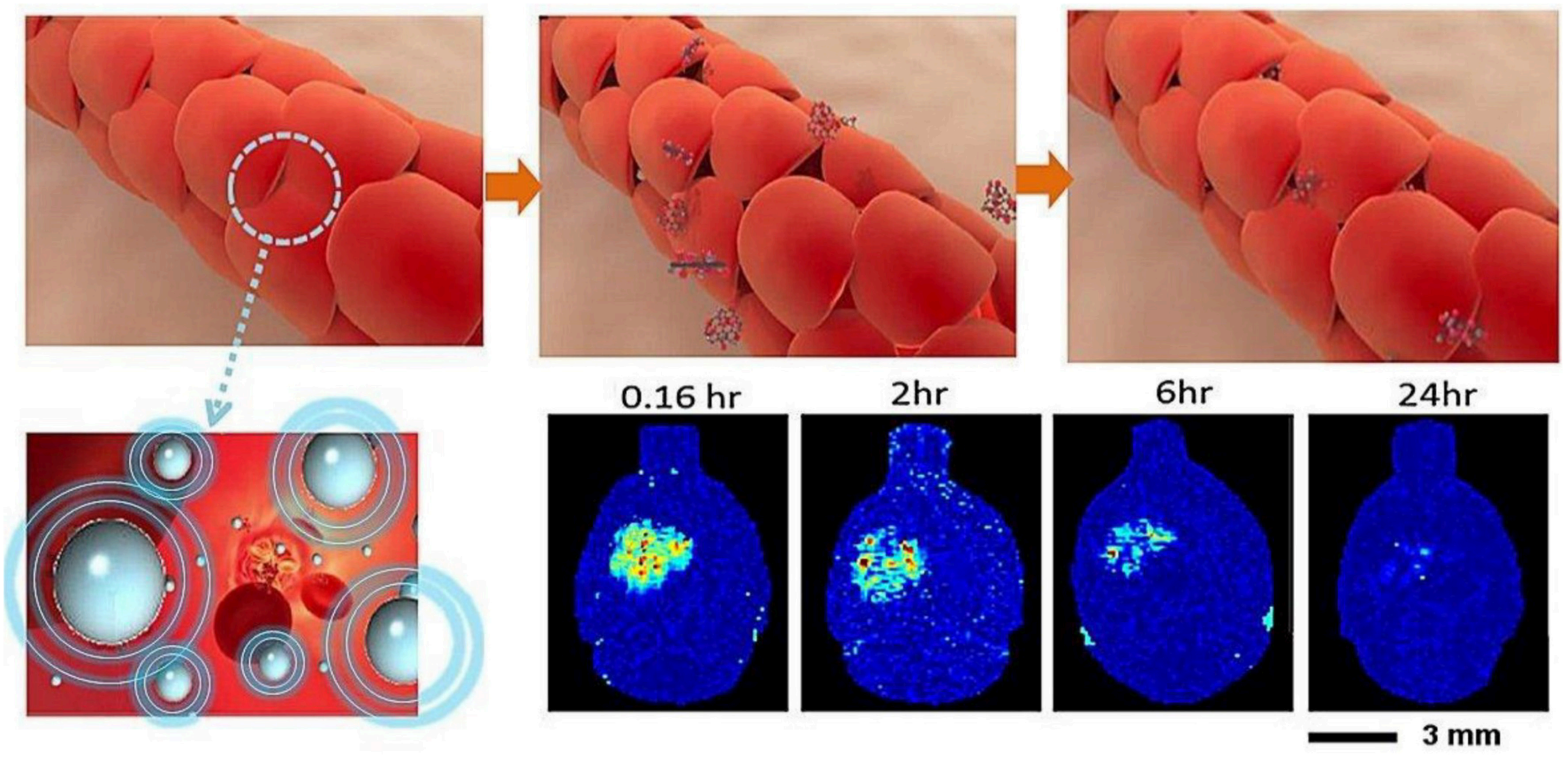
BBB opening concepts: interaction of microbubbles and focused ultrasound transiently disrupts the tight junction of the capillary lumen to allow therapeutic agents to penetrate into the brain. The BBB return to normal a few hours following focused ultrasound exposure (Chai et al., 2014).
